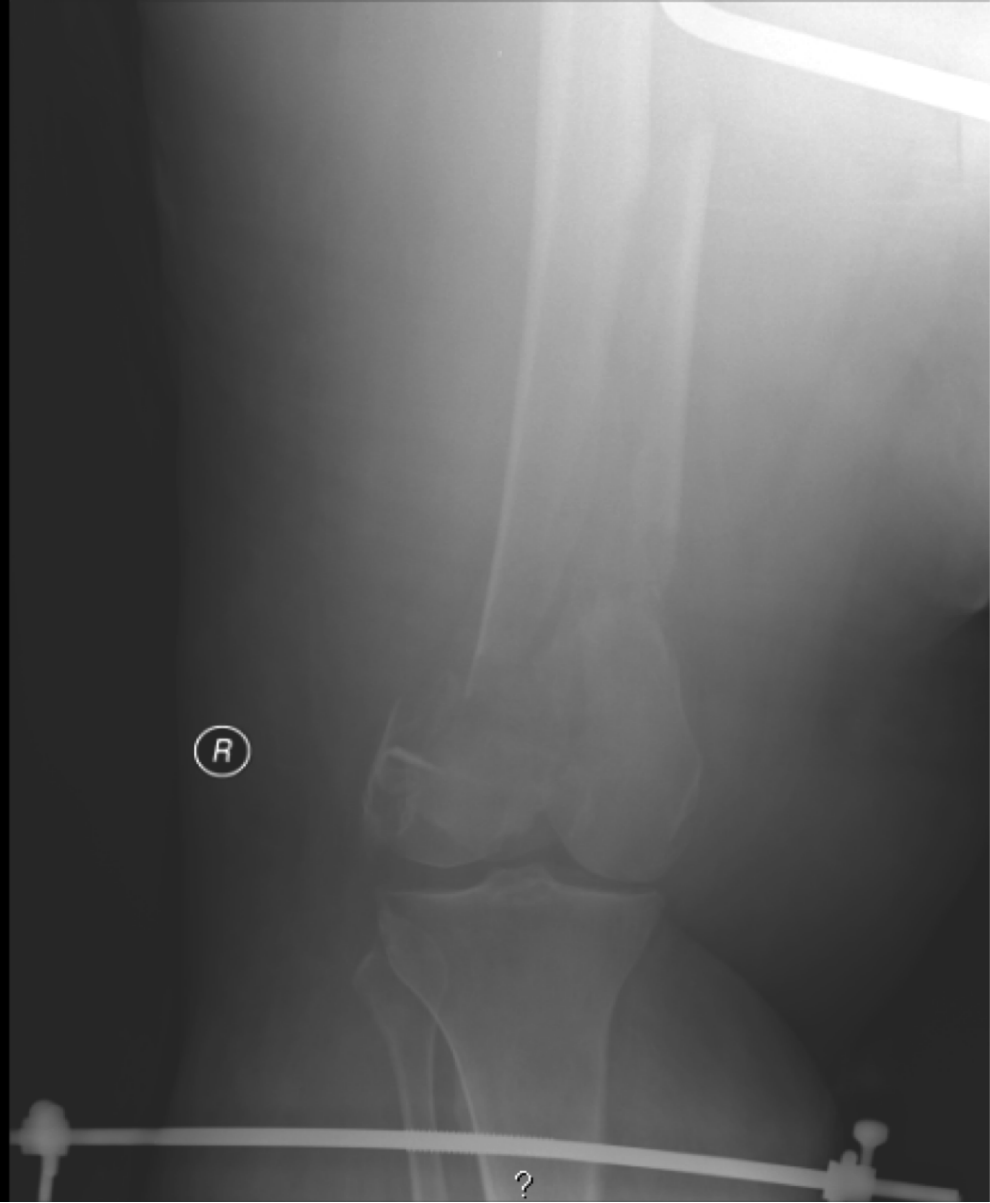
Posttraction radiograph of distal femur fracture with varus alignment.
